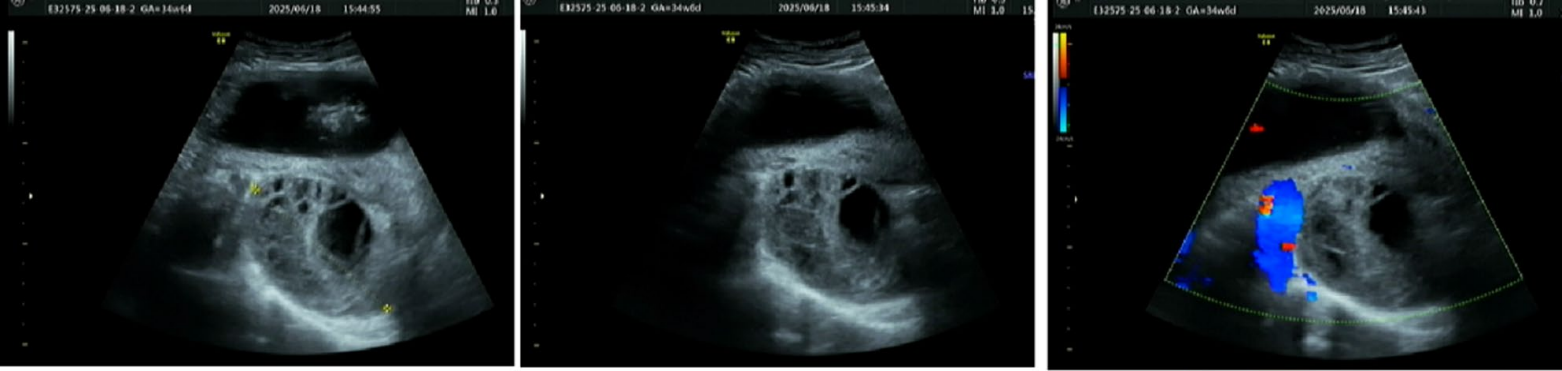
Transvaginal ultrasound at 34 + 6 weeks of gestation. The complex cystic mass posterior to the cervix has increased to 87 × 62 × 61 mm
